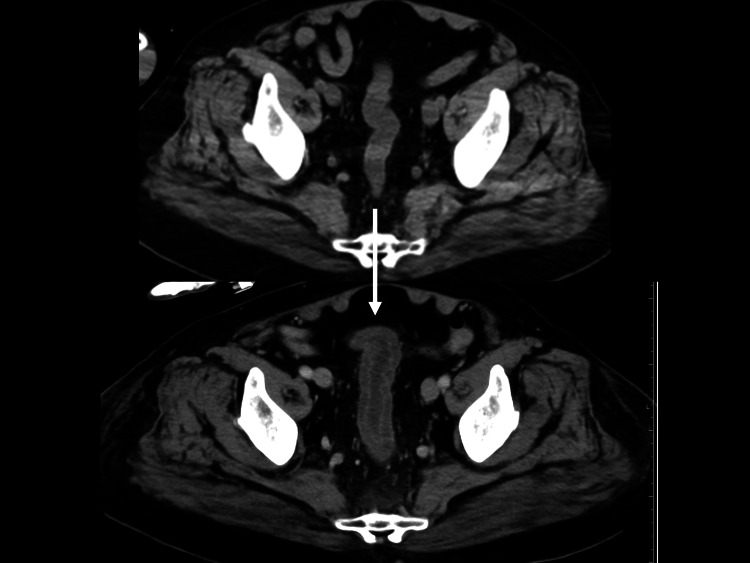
Abdominal computed tomography (CT) on arrival (upper) and day 3 (lower) CT showed thickening of the sigmoid and rectal colon, suggesting ischemic colitis. The arrow indicates the thickened sigmoid/rectal colon in the bottom image, with the top image showing the entity within normal limits.
